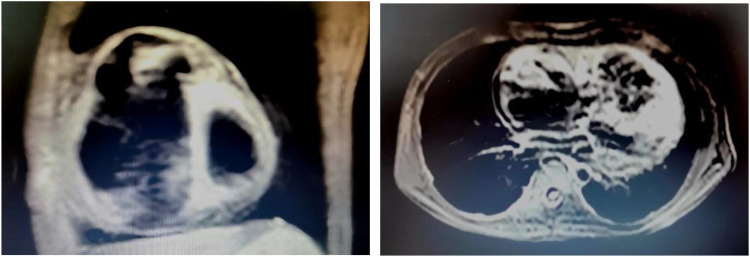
MRI results of the ARCM patient. MRI examination was performed 1 year after RFCA, at the time the recurrence of atrial flutter was detected in this child. At the same time, there was also an abnormal enlargement of the right ventricle and the presence of paroxysmal ventricular tachycardia. The result showed that the right atrium and right ventricle were significantly enlarged, the right ventricular wall was thinned, no exact abnormal fat signal was seen, and the right ventricular trabeculae were increased and thickened.
